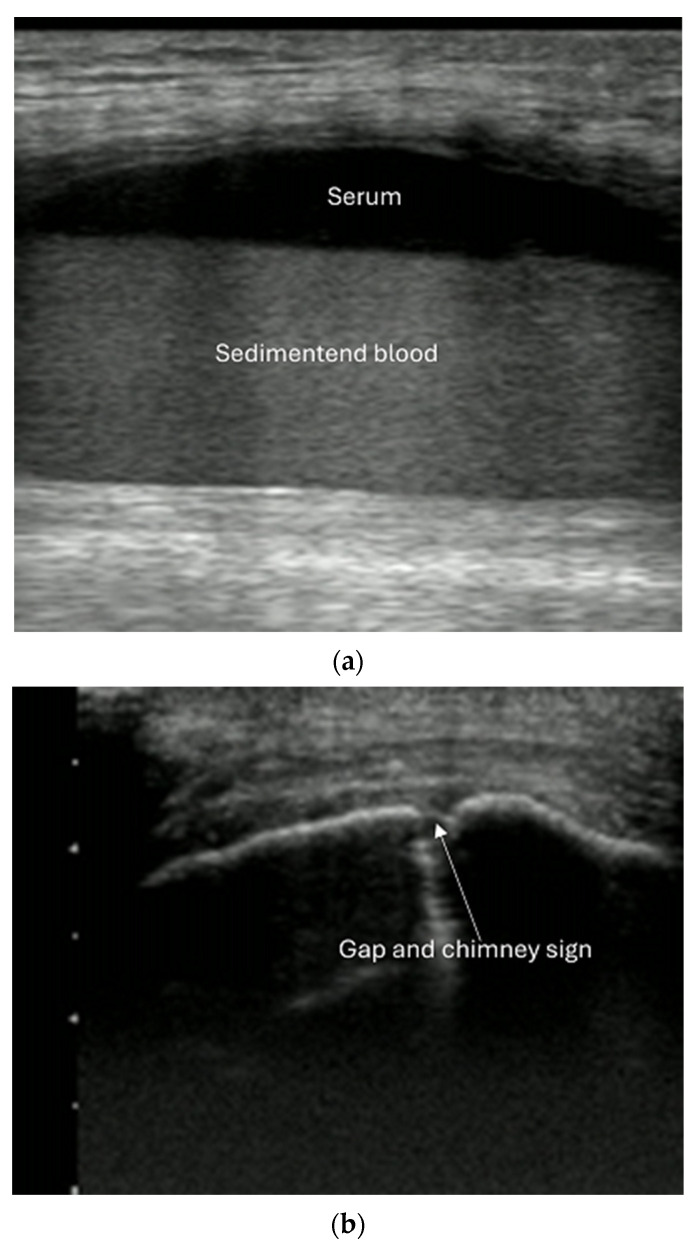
Occult fracture with negative X-ray. (a) Hemarthrosis of lateral knee recessus. (b) Lateral femoral condyle with gap and chimney sign.
